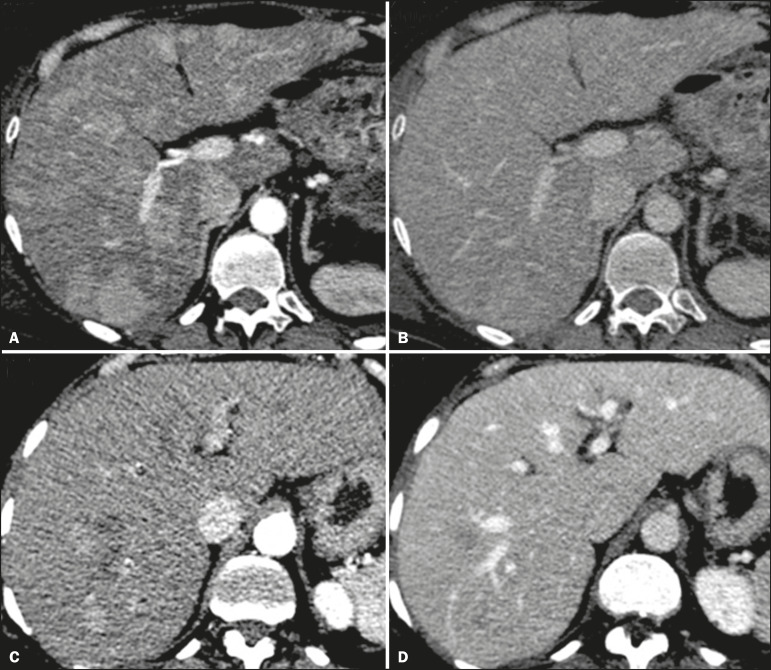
CT scans obtained in the arterial phase (A,C) and portal venous phase (B,D). Multiple liver nodules with a hypervascular enhancement pattern (A,C) and portal venous phase isointense attenuation in a case of a pancreatic NEN (A,B), and portal phase hypoattenuation in a case of an ileal NEN (C,D).
